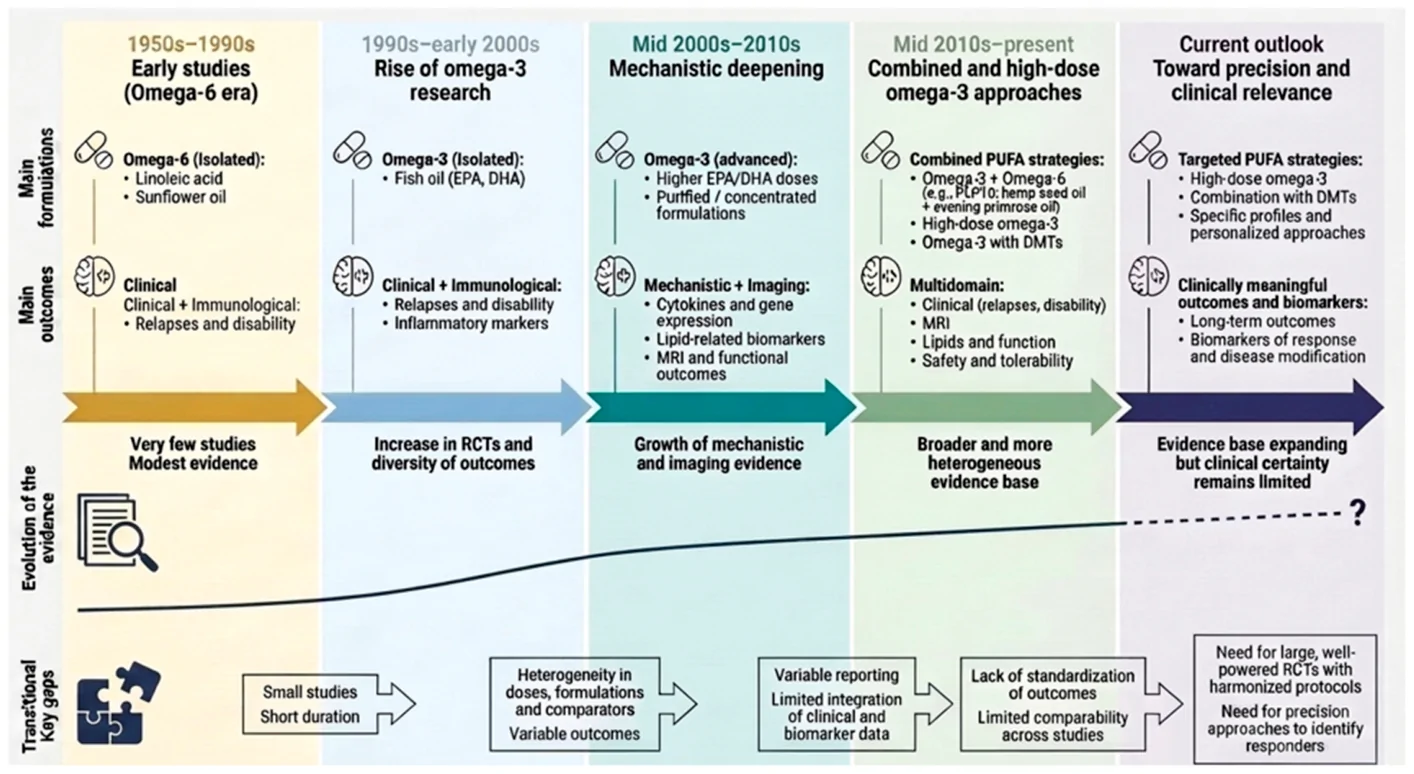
Chronological evolution of research on Polyunsaturated Fatty Acid (PUFA) supplementation in Multiple Sclerosis (created by the authors using NotebookLM image generation and subsequently refined for scientific accuracy). DHA: Docosahexaenoic Acid; DMTs: Disease-Modifying Therapies; EPA: Eicosapentaenoic Acid; MRI: Magnetic Resonance Imaging; RCTs: Randomized Clinical Trials.
